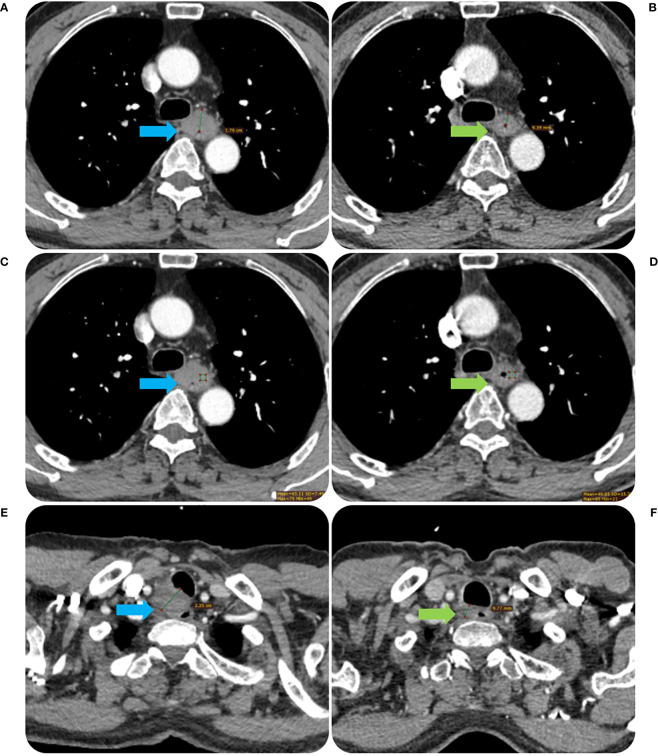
The contrast-enhanced CT images before and after RT of the same patient in Figure 2. The tumor MWT was 1.76 cm before RT treatment (A) and decreased to 0.94 cm after treatment (B). The CT value of the tumor was 65.11 Hu before RT treatment (C) and decreased to 48.85 Hu after treatment (D). The short diameter of the metastatic lymph node in the right proper supra tracheoesophageal sulcus was about 2.25 cm before RT treatment (E), which was reduced to 0.98 cm after treatment (F).
